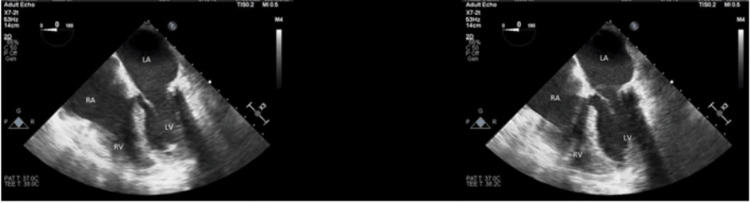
Mid-esophageal four-chamber view. A transesophageal echocardiogram was performed six hours after cardiac arrest, revealing moderately dilated right and left ventricles, biventricular systolic dysfunction, +1 mitral regurgitation, and akinetic septal wall. Left ventricular systolic function is severely reduced with an ejection fraction of 10 ± 5%.
